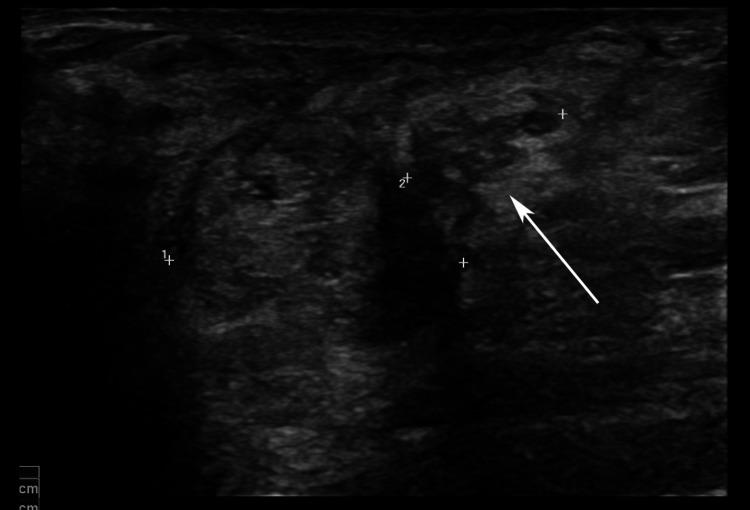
Ultrasound image 12 days after percutaneous core biopsy Ultrasound image 12 days after the biopsy showed two separate masses: the main mass at 12 o’clock and a further adjacent nodule at 2 o’clock in the sub-areolar location. A new 12-mm heterogeneous, cone-shaped nodule contiguous with the main mass was pointing medially and towards the skin (arrow).
